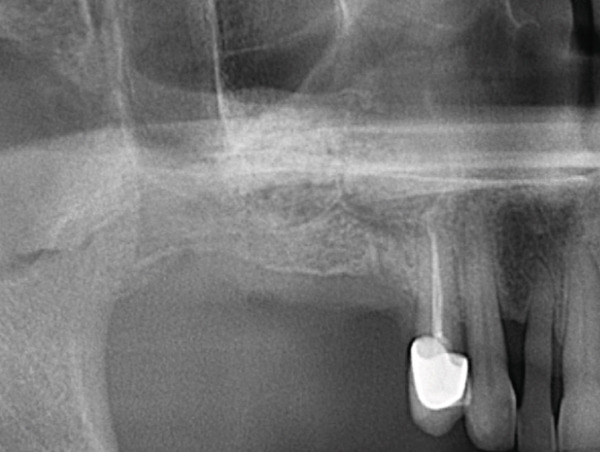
Panoramic radiograph 3 months postsurgery showing no apparent abnormalities.
